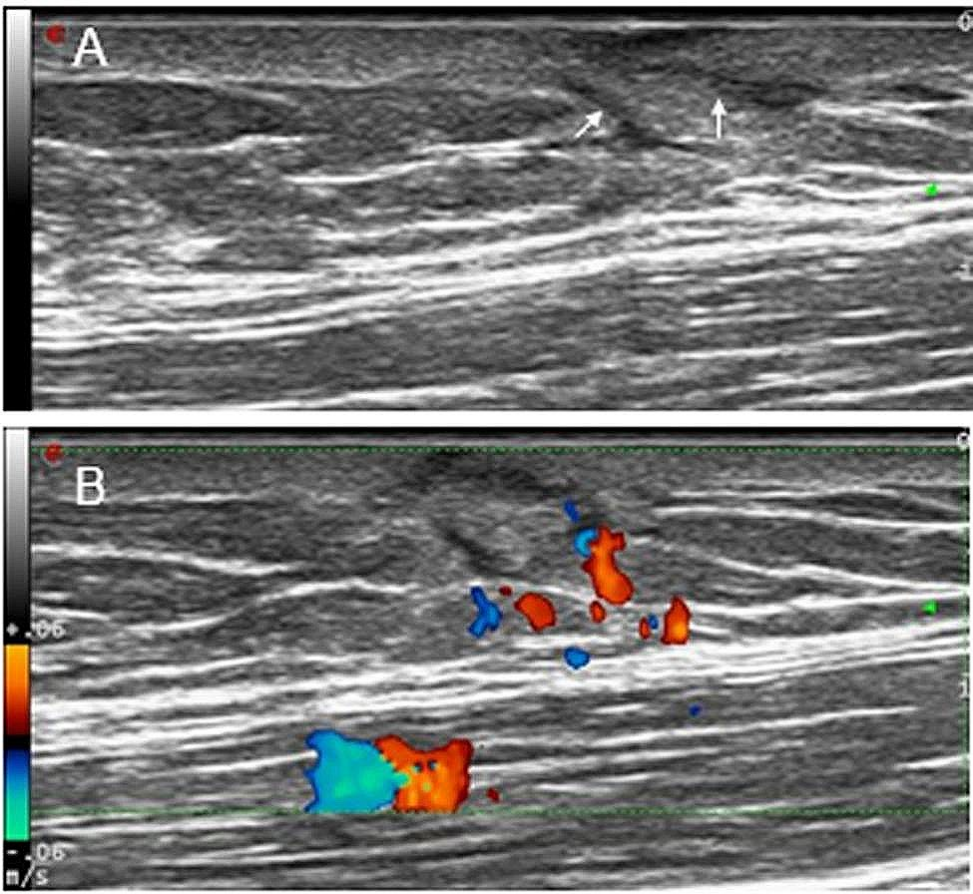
A A 32-year-old female with recurrent DFSP in the abdominal wall, transverse ultrasonogram revealing an ill-defined, hypoechoic, heterogeneous, and subcutaneous lesion, forming hypoechoic with finger-like projections (arrows). B Color Doppler ultrasonogram revealing rich blood flow signal
